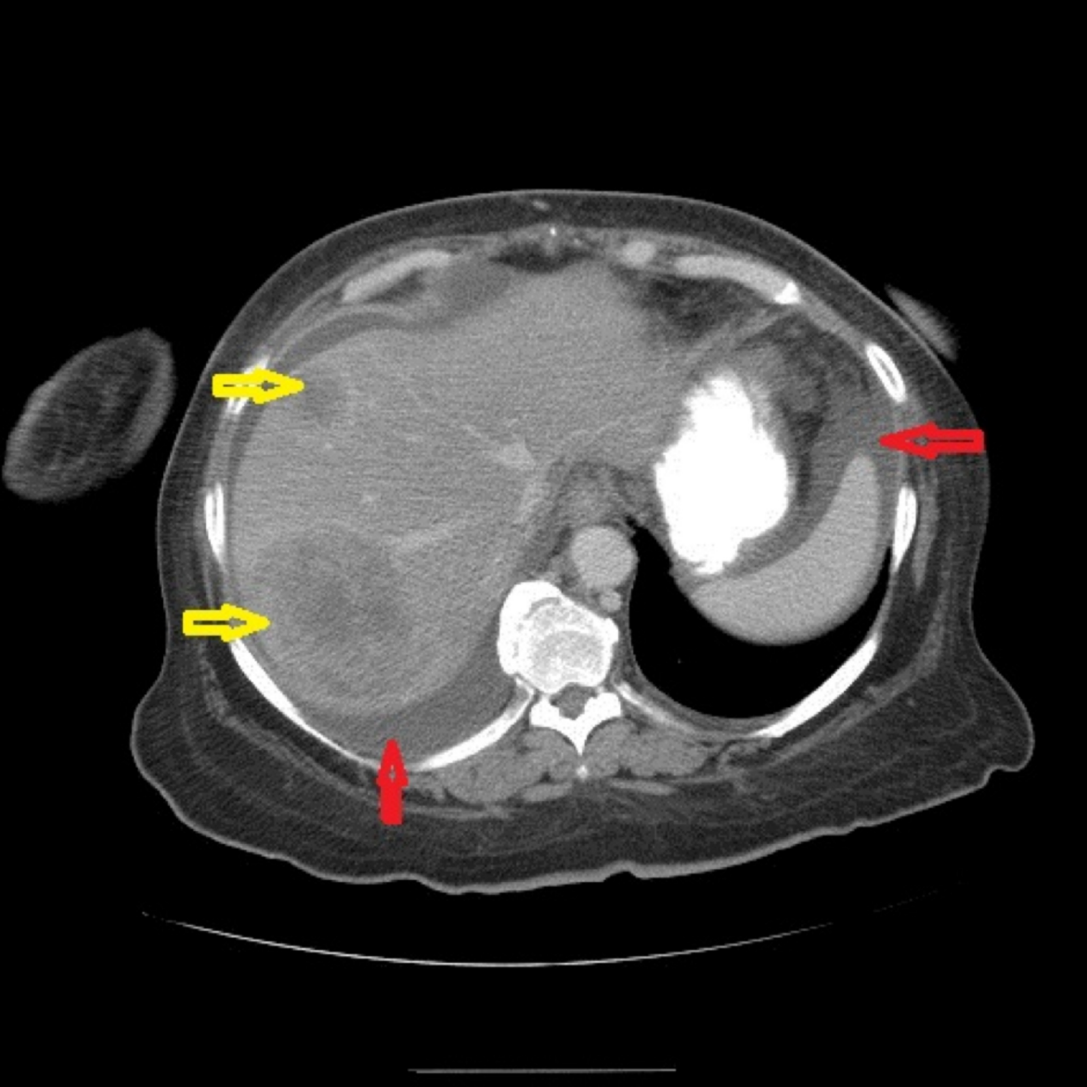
Repeat CT of the abdomen and pelvis in July 2018 - coronal view The image shows new and enlarged liver metastatic disease; the previous 48-mm metastatic lesion was later measured to be 76 x 66 mm as depicted by the yellow arrows. The red arrows show ascites CT: computed tomography
